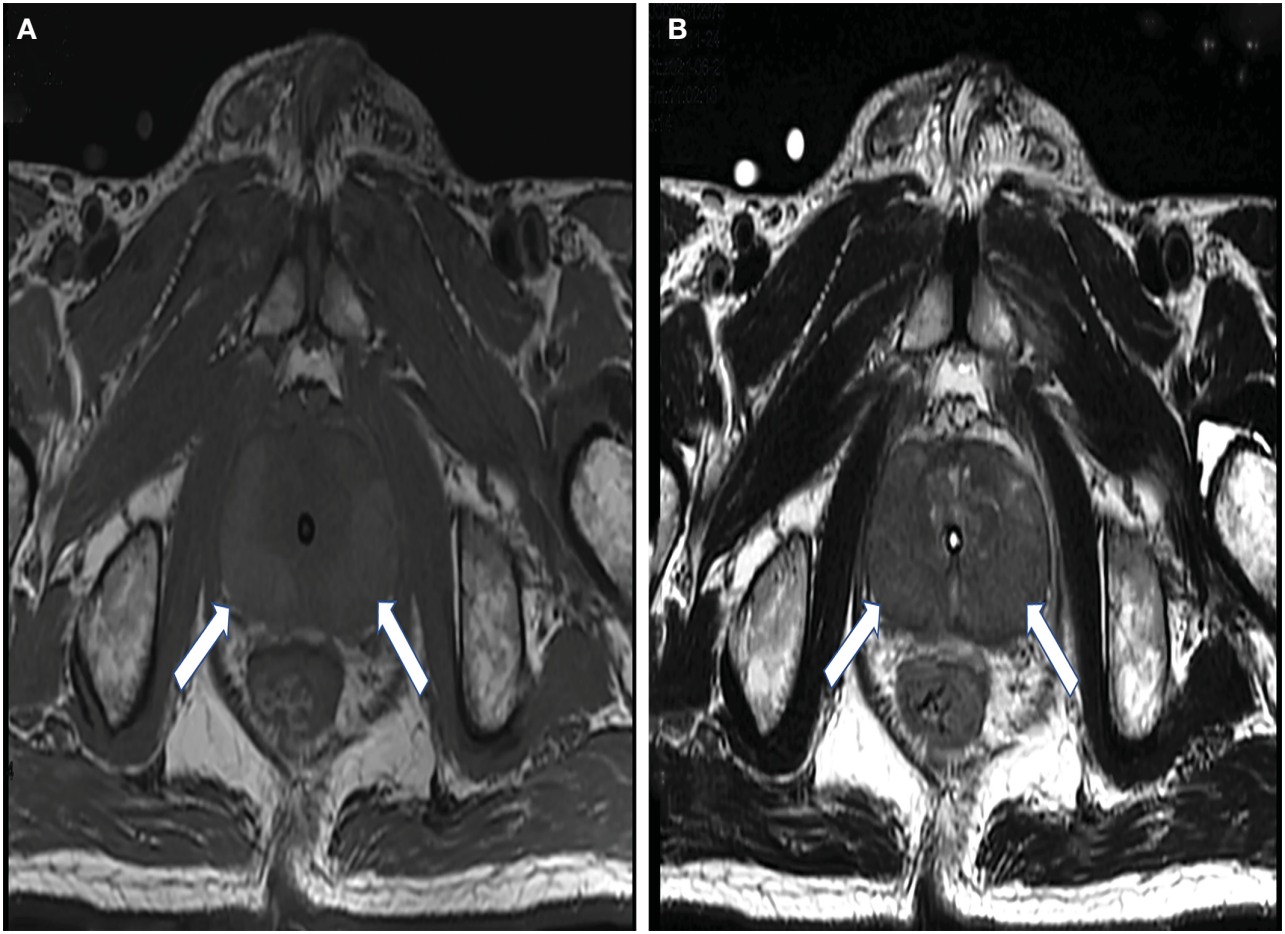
A 68-year-old man with elevated prostate-specific antigen (PSA). The lesions (*arrows*) showed a high signal on T1-weighted images (A) and a low signal on T2-weighted images (B).
